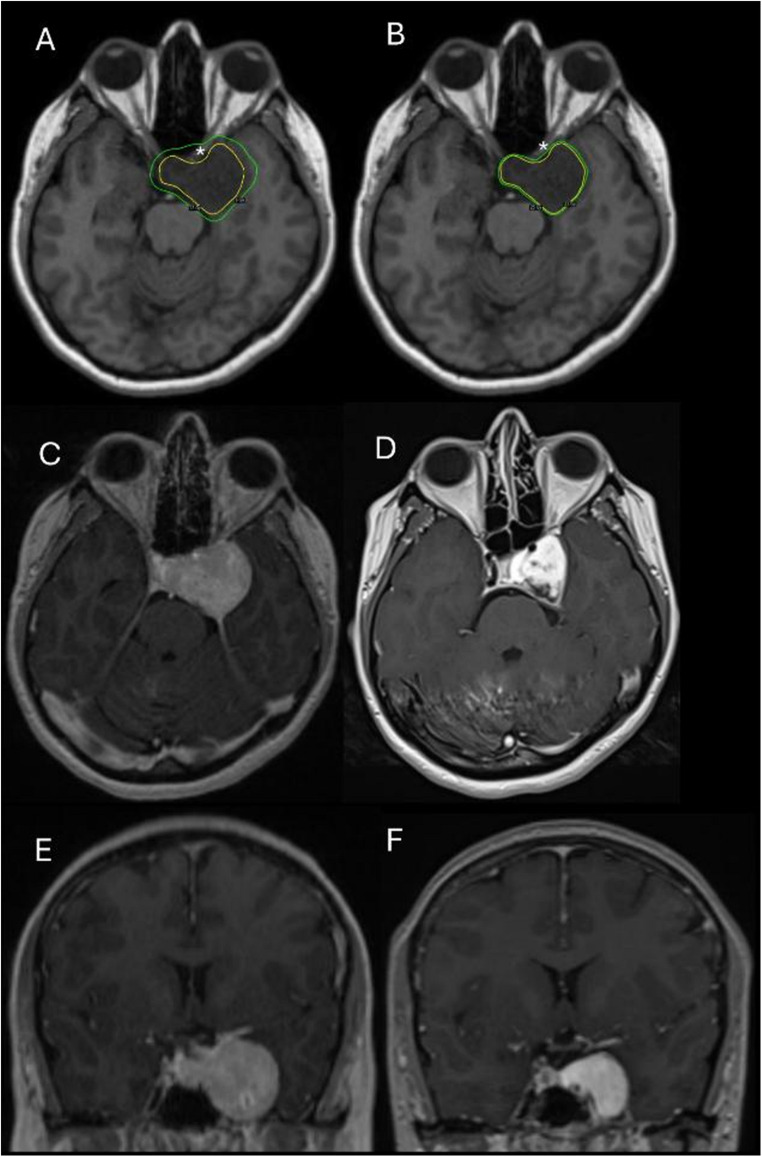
Representative imaging and treatment planning of Case 3 (Table 1), a 34-year-old female with a left CSH (21.4 cm³) presenting with CN III, IV, and V2–V3 deficits. (A) Hypothetical single-fraction plan delivering 12 Gy marginal dose (yellow) on axial non-contrast T1-weighted MRI: the 8 Gy isodose line (green), representing the single-fraction optic apparatus tolerance threshold, encompasses the left optic nerve (asterisk), precluding safe single-fraction treatment. (B) Actual hypofractionated plan delivering 25 Gy in five fractions: the prescription isodose (25 Gy, yellow) provides conformal target coverage, confirming adequate sparing of left optic nerve (asterisk). Axial (C) and coronal (E) contrast-enhanced T1-weighted MRI obtained on the day of treatment demonstrate a large left cavernous sinus mass. Corresponding axial (D) and coronal (F) contrast-enhanced T1-weighted MRI at 6-month follow-up show volumetric reduction of 24.3%
